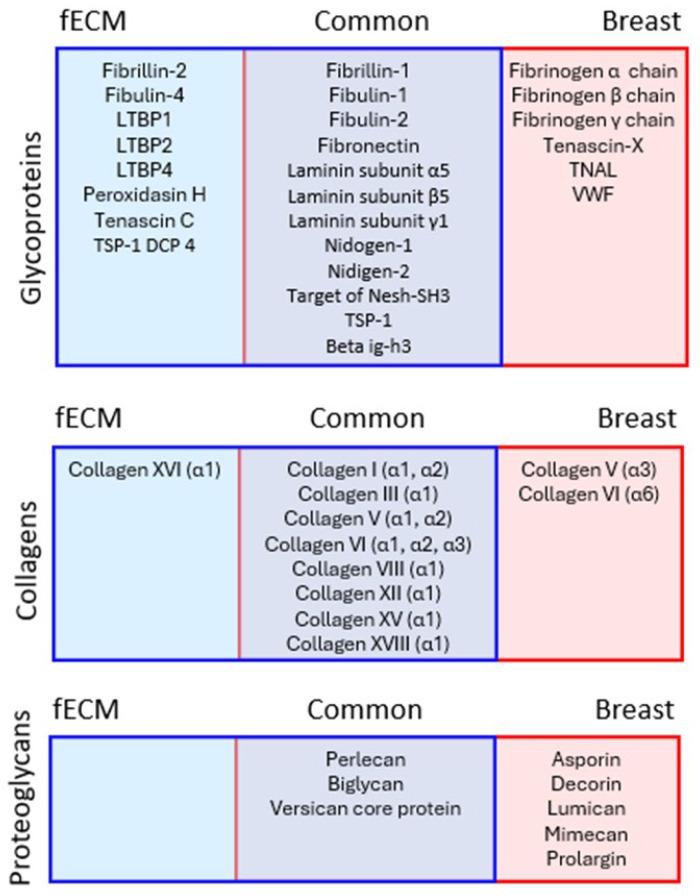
X-ray labile proteins in cultured fibroblast and breast tissue matrices. Proteins found only in fECM, only in breast tissue, or in both, that were identified by PLF analysis as structurally compromised by X-ray exposure. Each of these proteins contained at least one bin within their protein sequence with a statistically significant change in peptide MS1 intensity after exposure to 50 Gy or 100 Gy of X-ray exposure.
